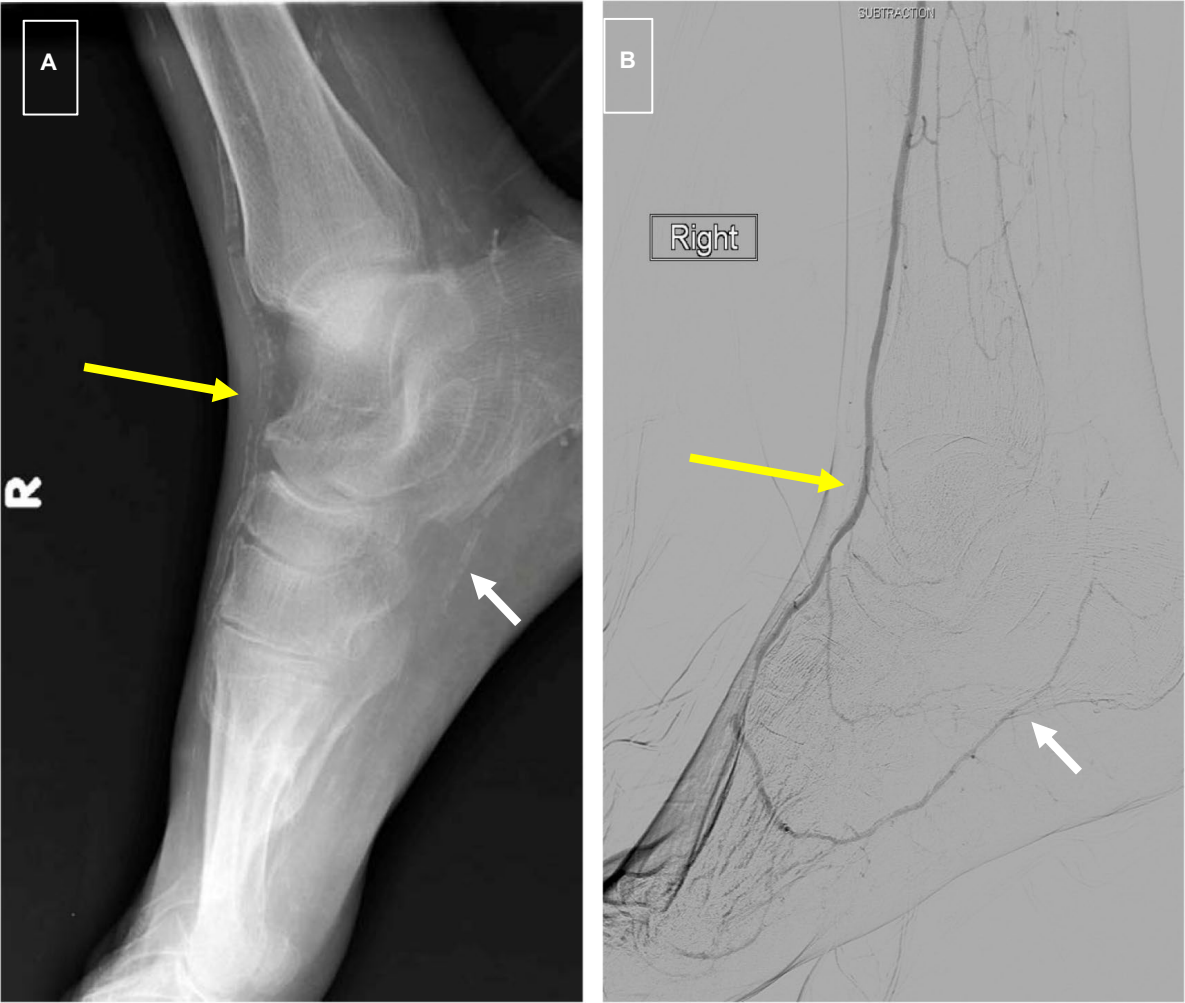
(A) Foot x-rays showing typical “railroad track” appearance of dorsalis pedis artery signifying medial calcification (long yellow arrow) and “patchy” appearance of intimal calcification in plantar artery (short white arrow). (B) Digital subtraction arteriogram from same patient showing a patent DP (long yellow arrow) as is typical of medial calcification and high-grade stenosis of plantar arteries associated with intimal calcification (short white arrow).
